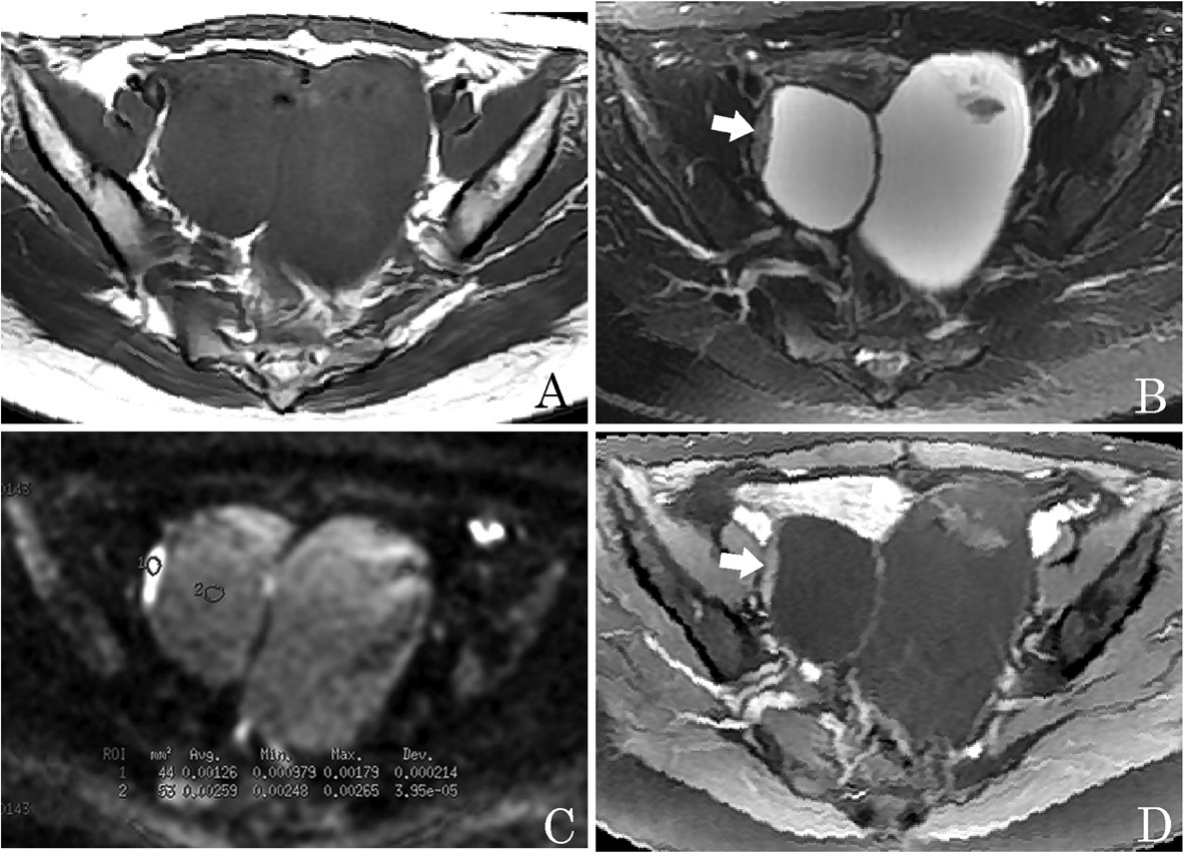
A 28-year-young woman with bilateral benign serous ovarian cystadenomas. (A) An axial T1-weighted image shows a pelvic tumor with hypointensity. (B) Axial T2-weighted image shows a complex adnexal tumor with solid and cystic components, and solid components as vegetations with intermediate signal intensity (arrow). (C) Axial diffusion-weighted imaging (DWI) obtained at b =1,000 s/mm^2^ shows the solid component as hyperintense with a high apparent diffusion coefficient (ADC) value (circle 1, ADC = 1.26 × 10^-3^ mm^2^/s), and the cystic component with an even higher value (circle 2, ADC = 2.59 × 10^- 3^ mm^2^/s). (D) Axial contrast-enhanced T1-weighted image demonstrates slight enhancement of the solid component (arrow).
